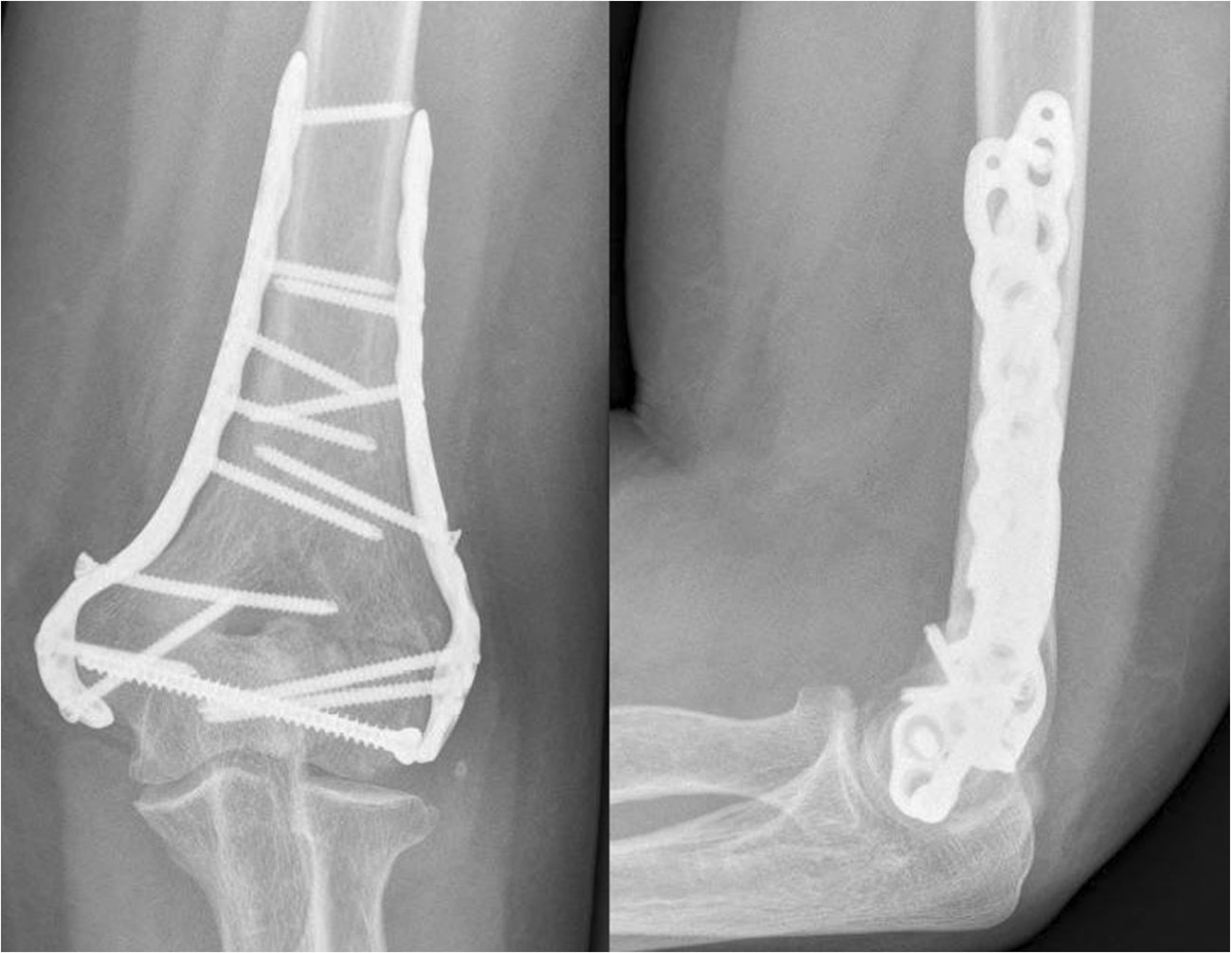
Anteroposterior and lateral elbow radiographic views of 180° parallel plating of a distal humerus fracture
